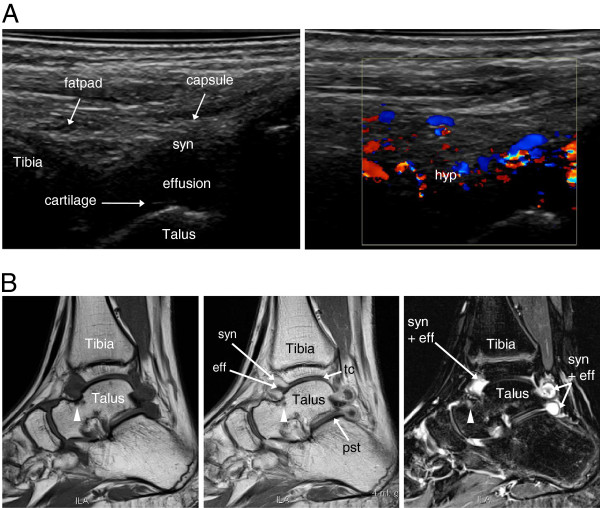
Imaging of the symptomatic ankle region of an 11-year-old girl with JIA. (A) Sagittal US scanning of the anterior talo-crural joint showing synovial hypertrophy (syn) to the left and hyperemia (hyp) on color Doppler to the right. (B) T1-weighted sagittal MRI images before (left) and after (middle) contrast injection. The left image reveals a small bony erosion at the neck of the talus (arrowhead). The erosion is surrounded by bone marrow edema (arrowhead, middle image). In the same image synovial hypertrophy with contrast enhancement (syn) and effusion (eff) are visualized in the anterior and posterior recesses of the talo-crural (tc) and posterior subtalar (pst) joints. On the STIR image to the right, high signal intensity depicts the location of synovitis (syn + eff) and the bone marrow edema surrounding the erosion (arrowhead, right image).
